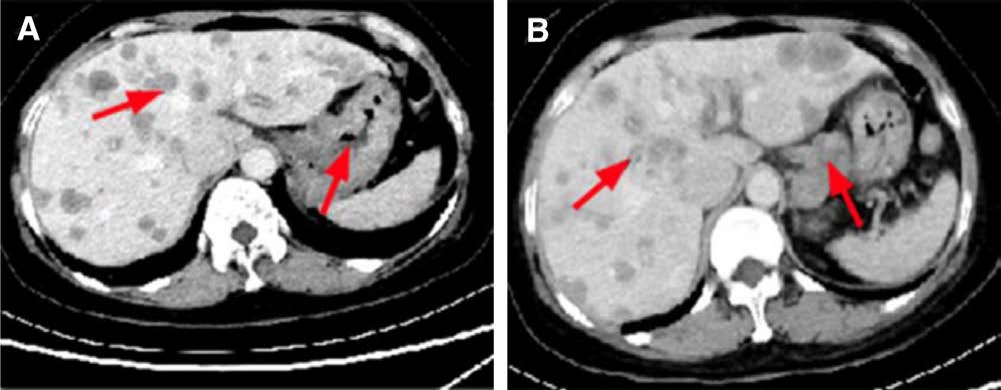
Abdominal enhanced CT. (A) Thickening of the stomach wall, a tumor with multiple metastases to the liver (arrow). (B) Multiple metastases to the liver and lymph node metastases to the side of the lesser curvature of the stomach (arrow).
